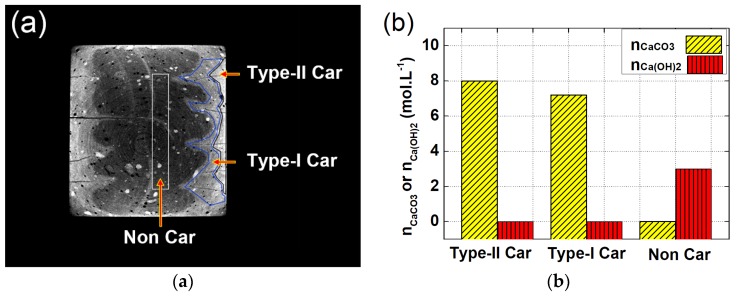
Sampled areas and experimental results for TGA: (a) selection of the sampling areas based on rendered CT slice; (b) measured CH and $C\bar{C}$ concentration from variant local areas.
